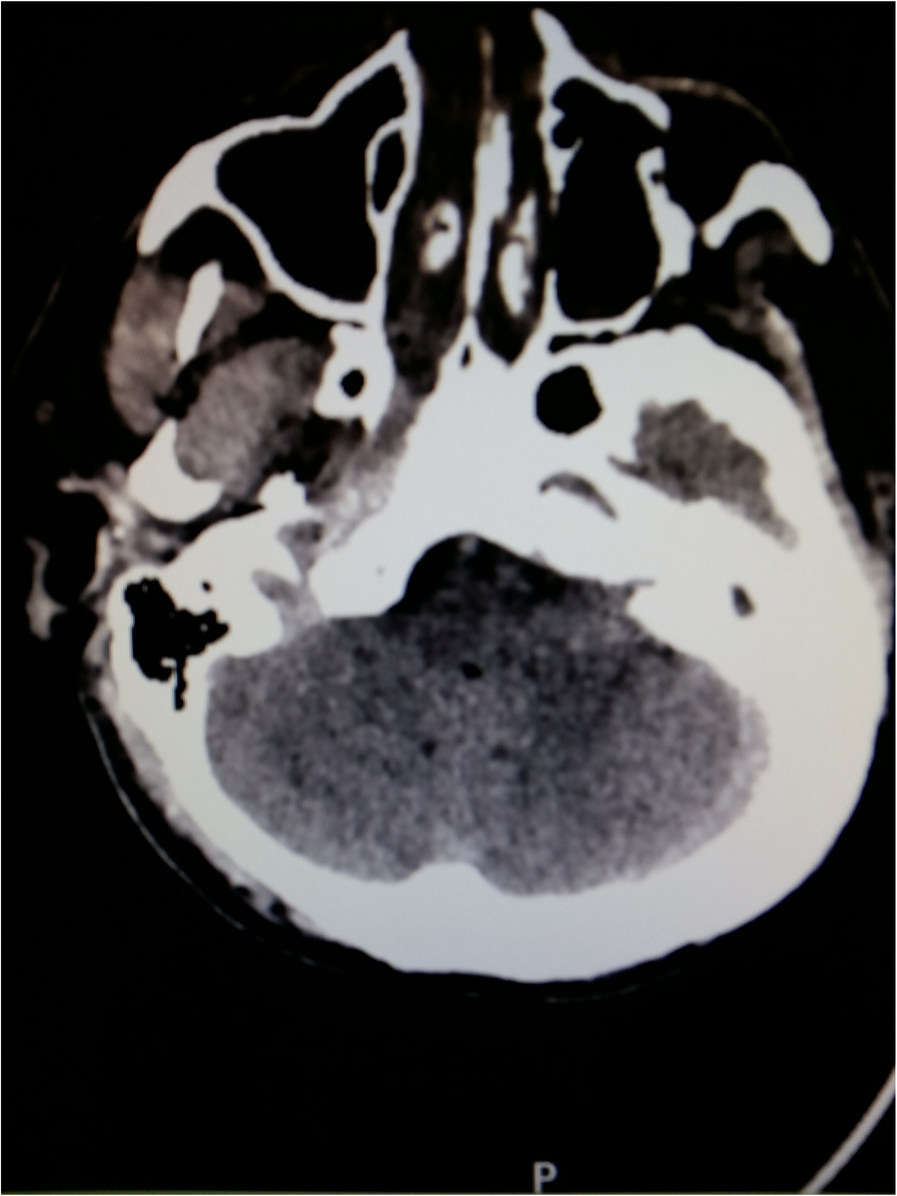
Skull CT: horizontal slice of brain with left side mastoiditis
